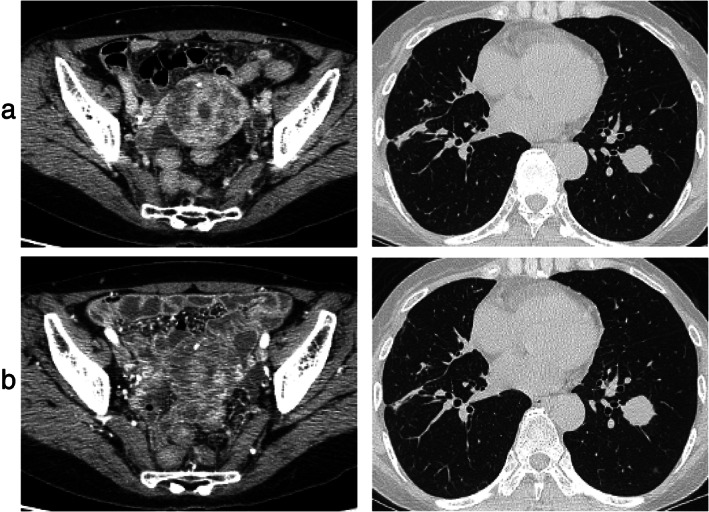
Lower abdominal and chest computed tomography findings at recurrence during alectinib therapy in the present case. (a) Uterus mass (arrows) and pulmonary mass (primary lesion in right lung and metastatic lesion in left lung). (b) Uterus and pulmonary mass after treatment with ceritinib in the present case
